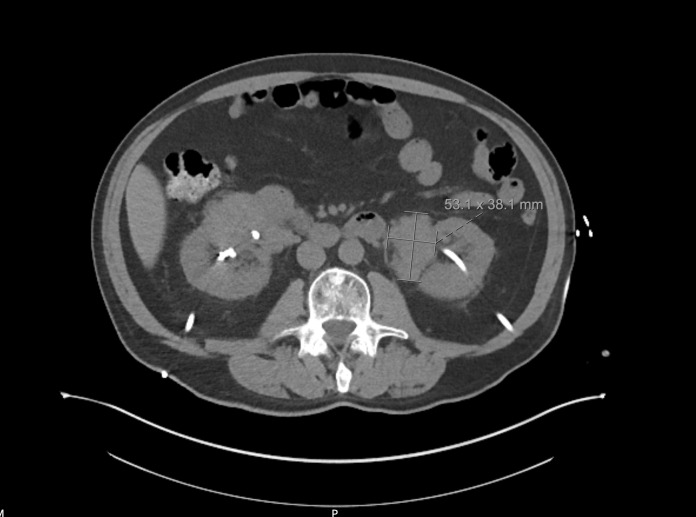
Computed tomography, coronal view in the abdomen demonstrating interval placement of bilateral percutaneous nephrostomies. On the left the plasmacytomas measure 5.3 cm in maximum diameter.
